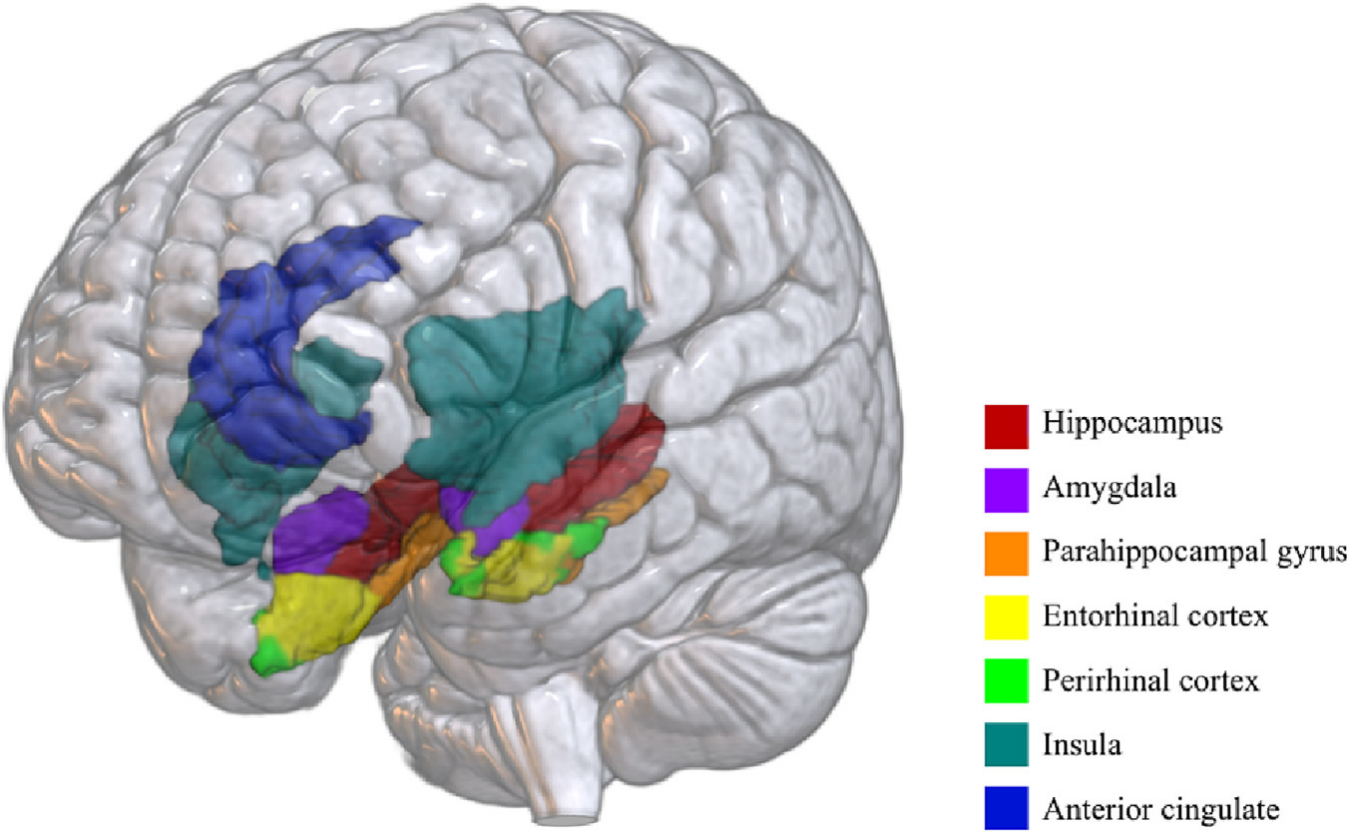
ROIs An example of individually segmented ROIs in a younger adult participant. ROIs were defined using automatic segmentation by FreeSurfer ver.6 on a T1-weighted MRI. Abbreviations: ROI = region-of-interest.
